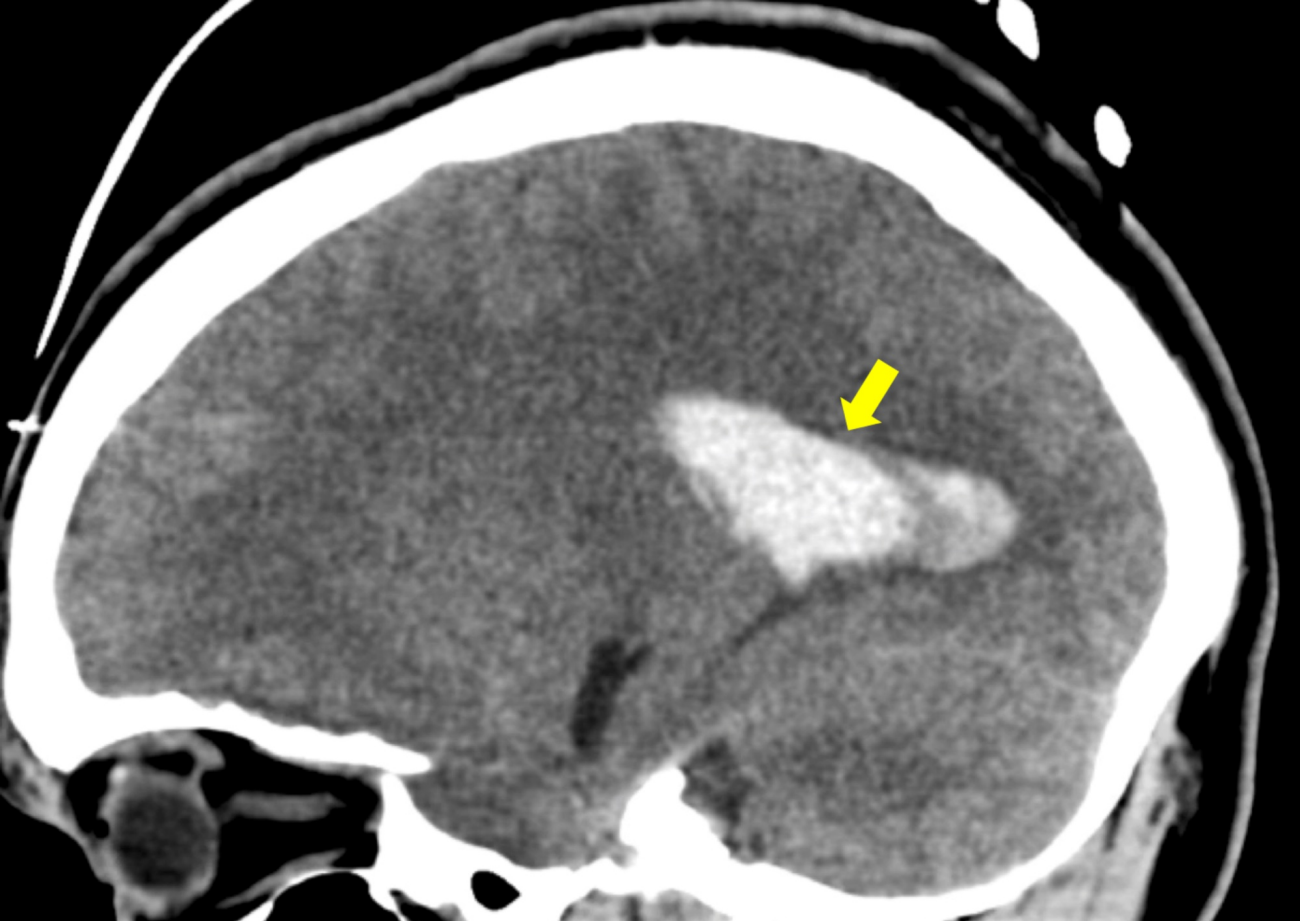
Sagittal CT head showing left intraventricular hemorrhage CT, computed tomography
